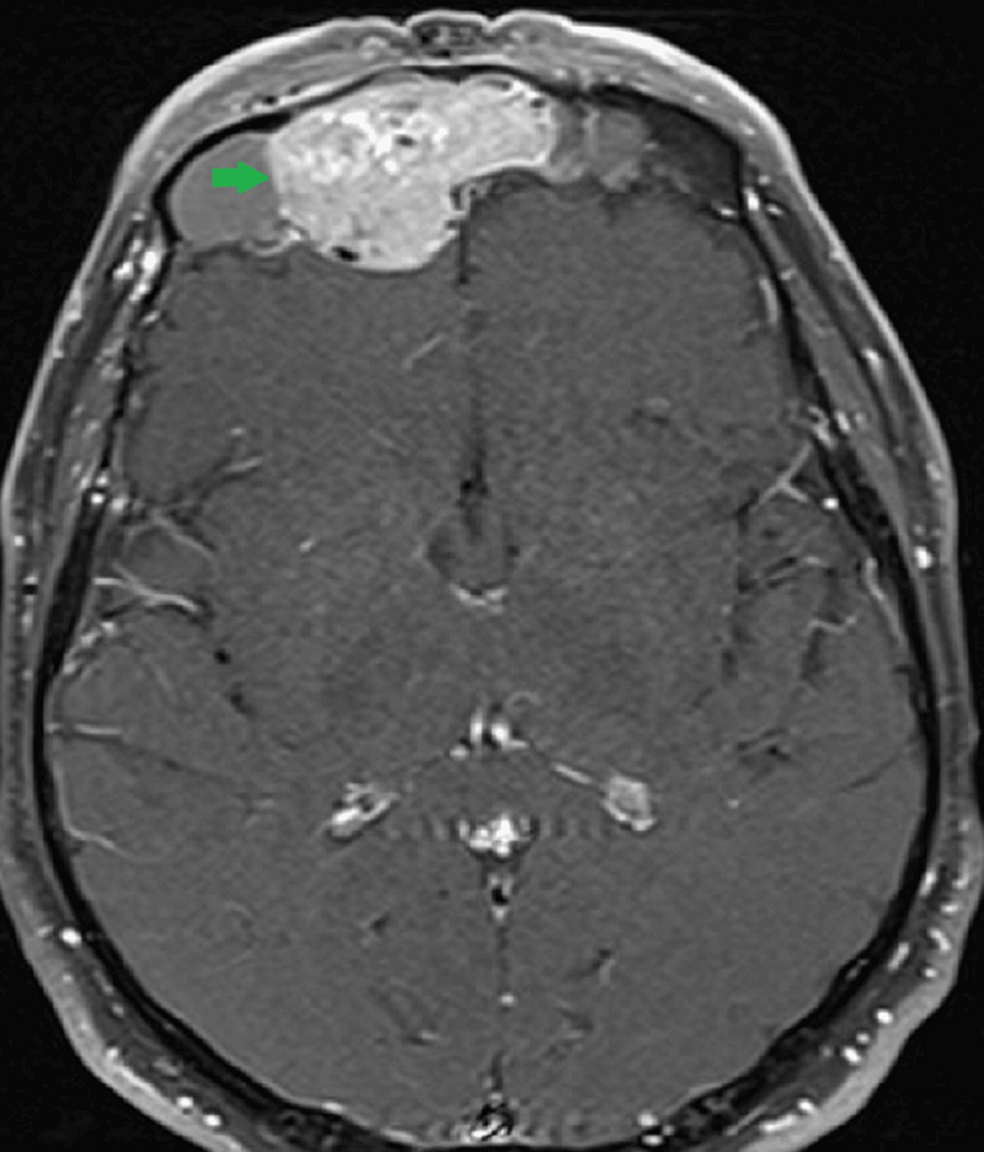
MRI brain (axial, T1 post-contrast) Large enhancing mass in the right frontal sinus (green arrow) destroying the posterior wall of the right frontal sinus with extension in the anterior cranial fossa, closely abutting and compressing the frontal lobe.
